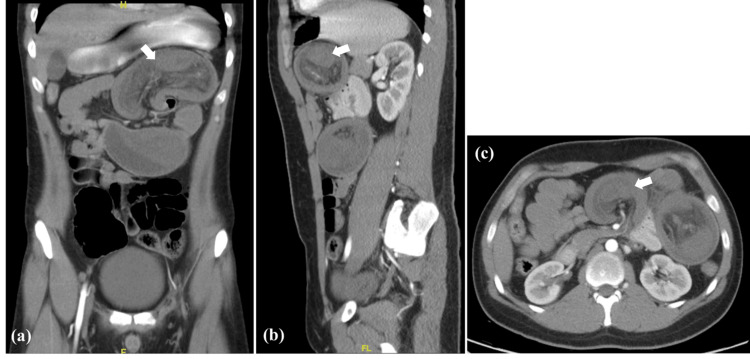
CT scan with oral and IV contrast: (a) coronal view, (b) sagittal view, (c) axial view, all showing dilated proximal jejunal loops (arrows) at the left upper abdomen with configuration of bowel within bowel, indicating the possibility of jejunojejunal intussusception. Congestion of the adjacent mesentery is also present with no passage of oral contrast beyond the intussusception point.
